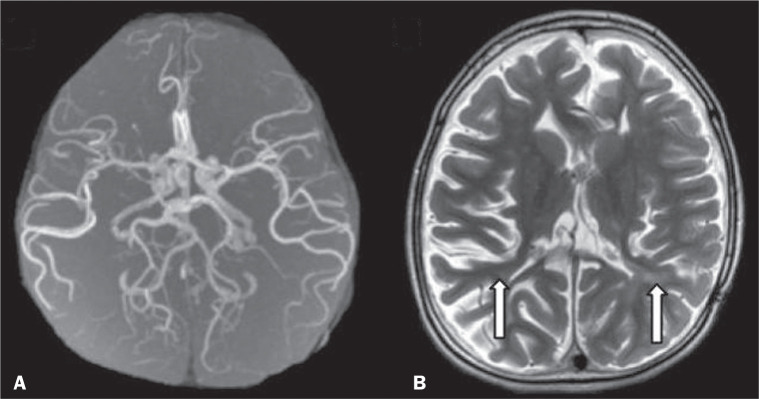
Vascular abnormalities in INMDs. MRI scans. A: Three-dimensional
